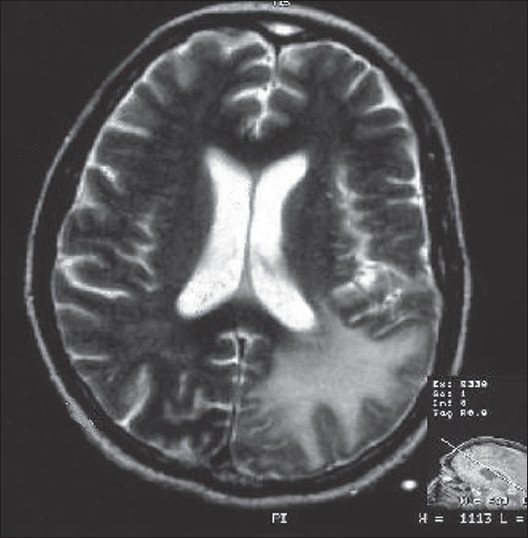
Cranial MRI, T2 sequence shows hyperintense subcortical lesion in left parietooccipital region in a patient with chronic lymphocytic leukemia, following fluderabine therapy
